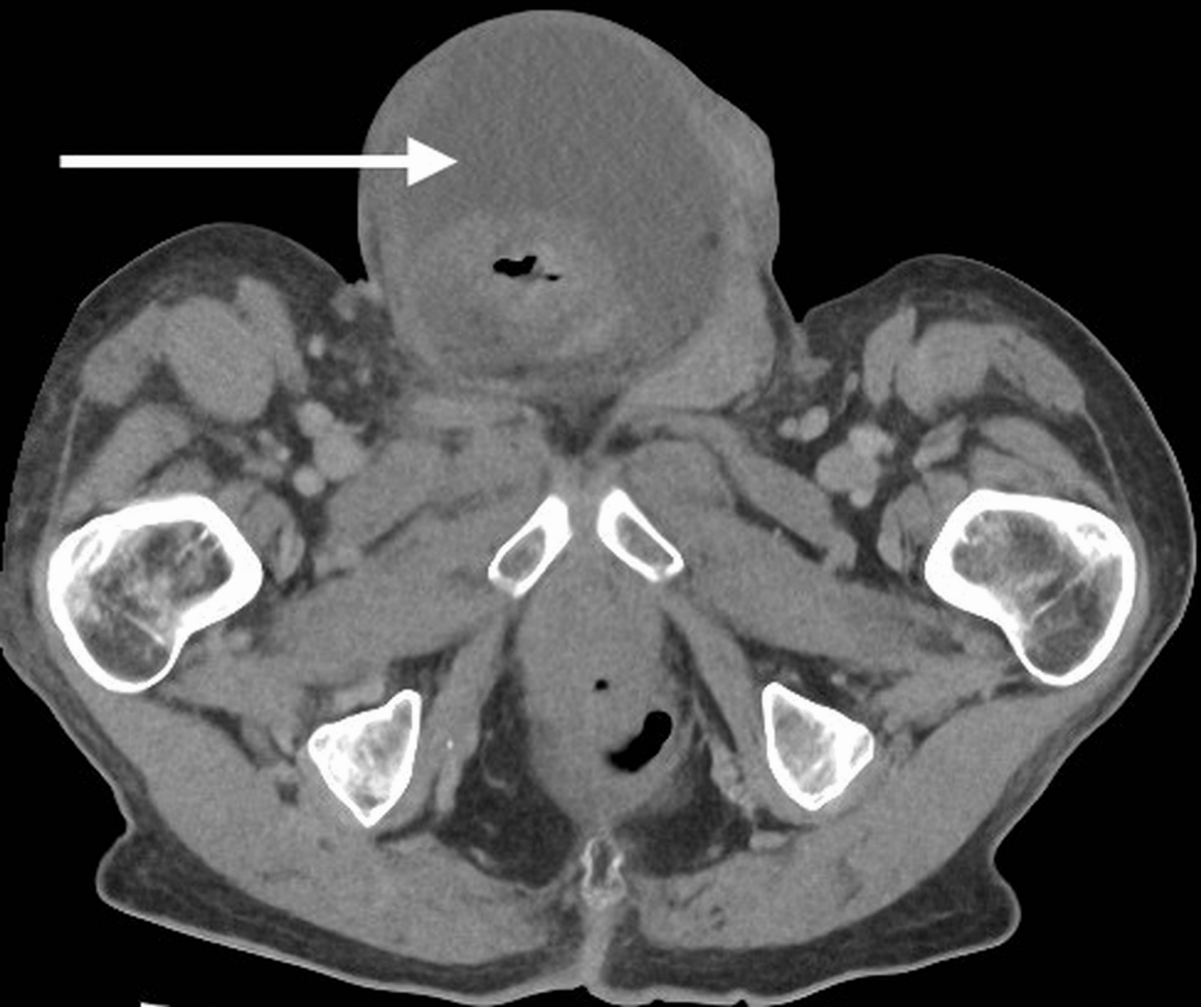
CTAP axial plane - 2 The hydrocele CTAP: computed tomography of the abdomen and pelvis
